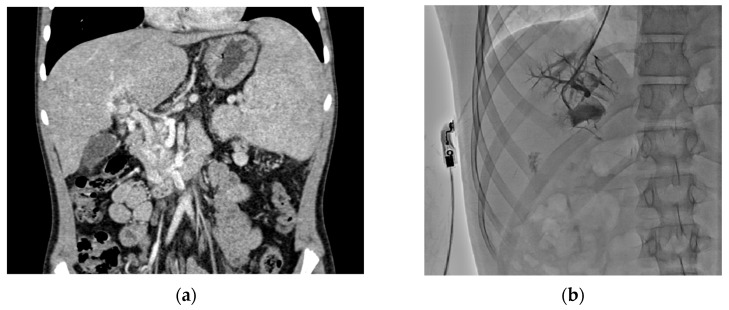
Preoperative imaging: CTA image (a) visualizing cavernous transformation of the portal vein with collateral vessels and retrograde portography (b) obtained via jugular vein catheterization and visualizing patent intrahepatic portal veins.
